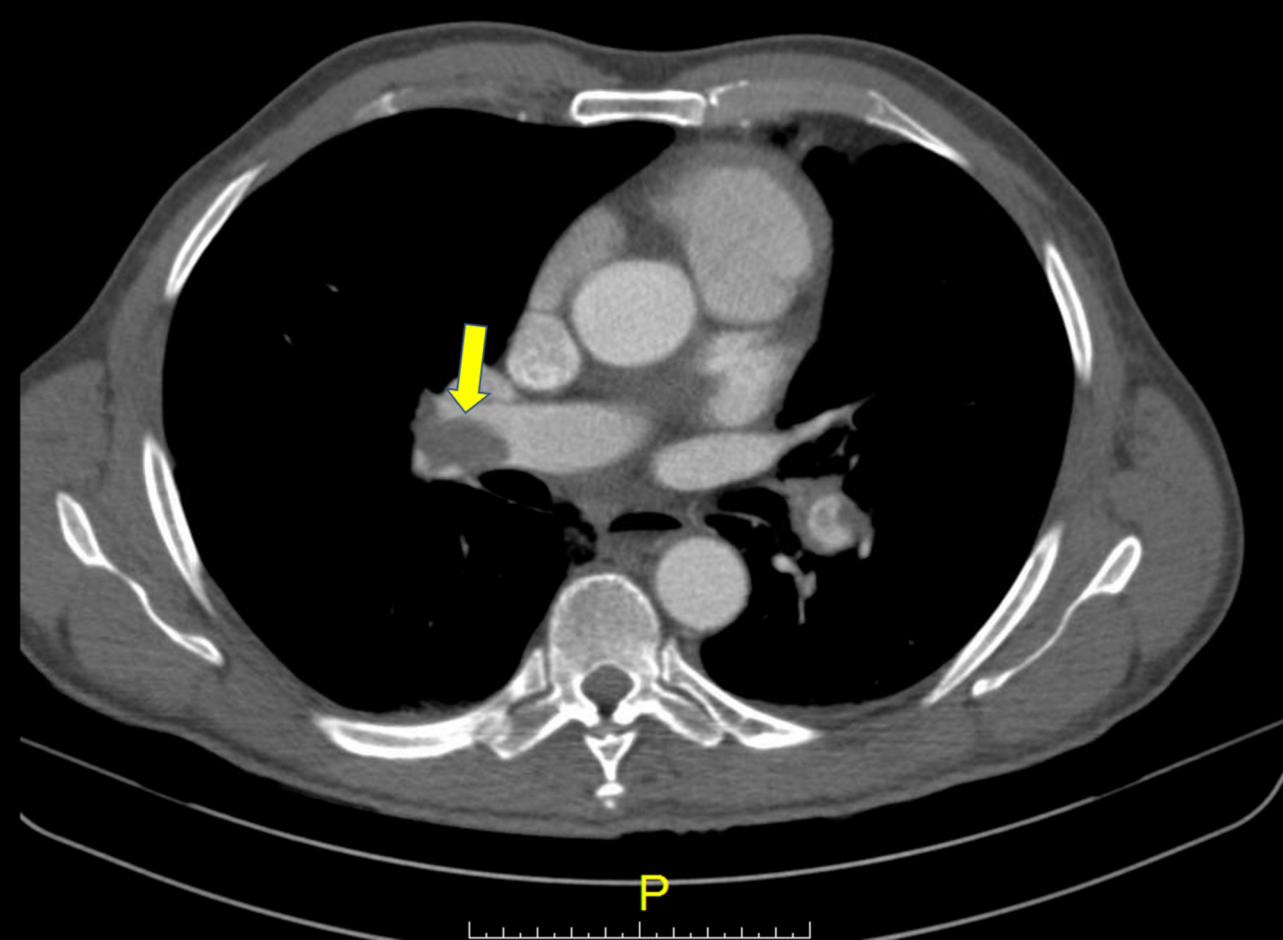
CT angiogram of the chest showing thrombus in the right pulmonary artery (yellow arrow)
